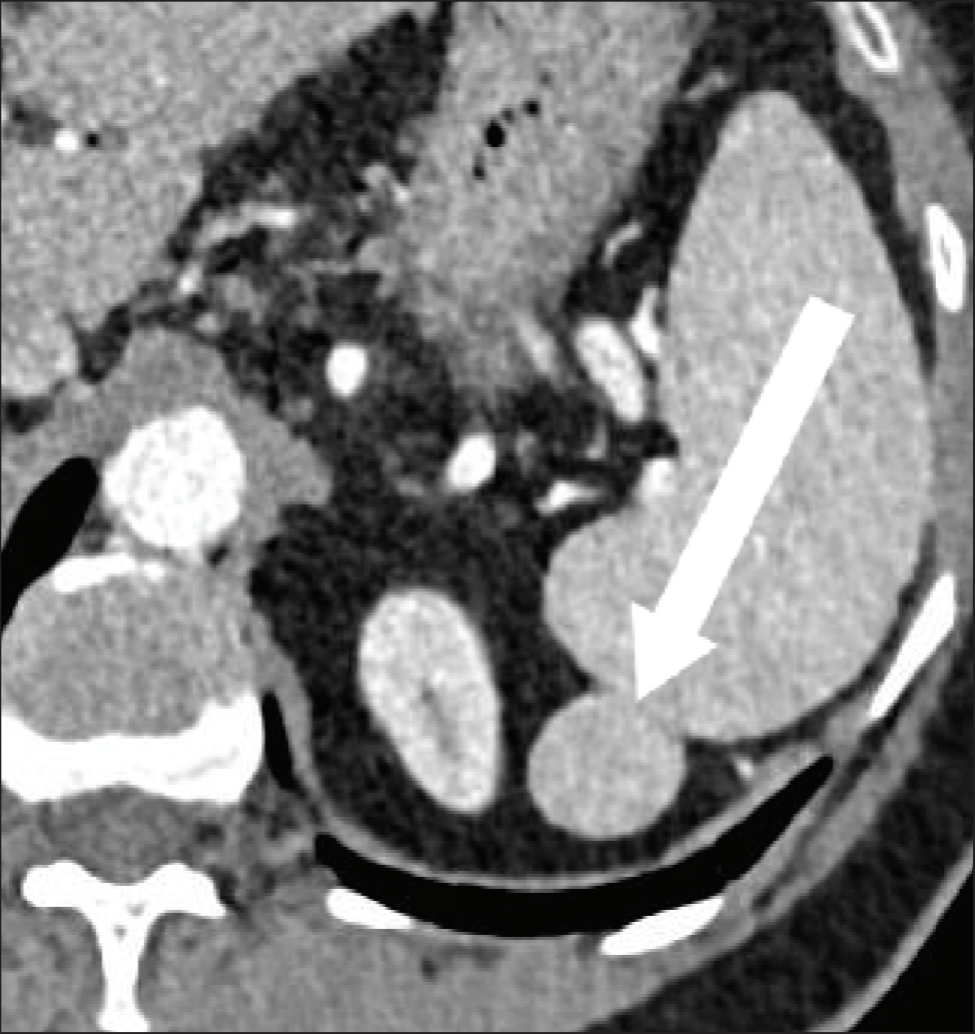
Axial enhanced CT slice: the WAS (arrow) between the upper renal pole and the spleen.
